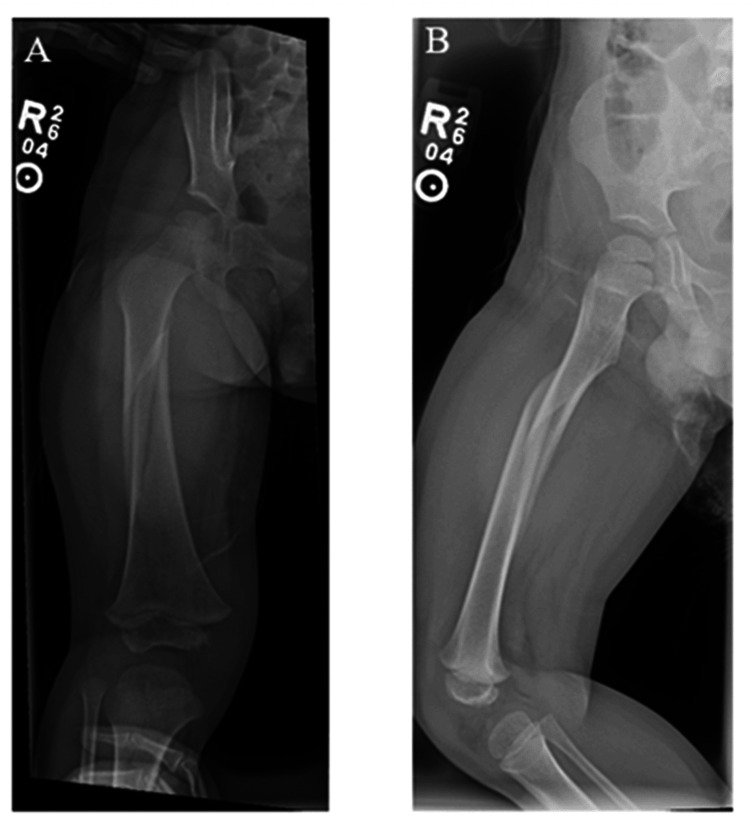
Initial imaging for case 3, including (A) AP and (B) lateral radiographs of the right lower extremity demonstrating an oblique subtrochanteric femoral shaft fracture
